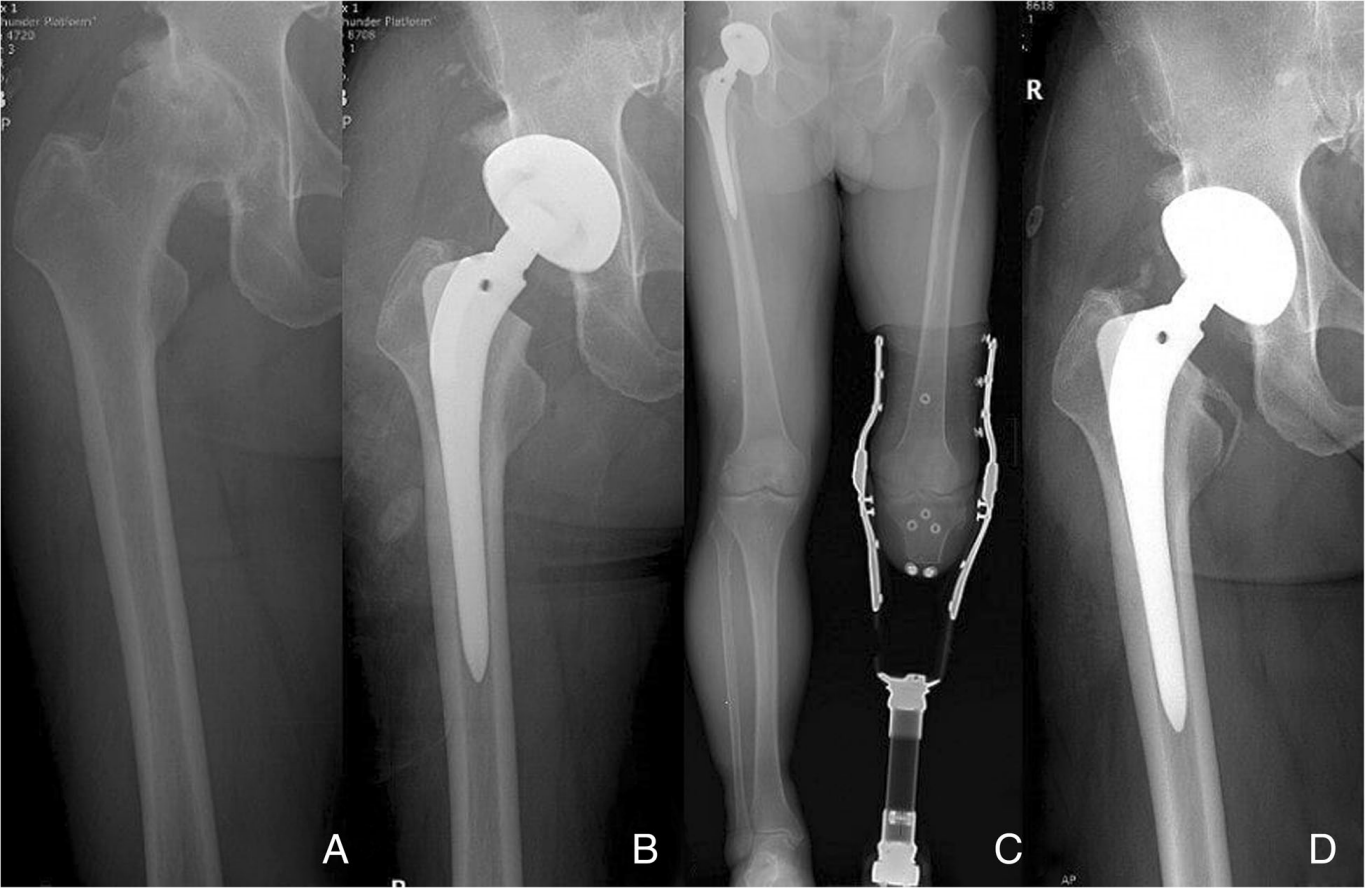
a Preoperative radiograph of a 67-old-year man with primary osteoarthritis of the hip joint. b Postoperative radiograph shows the excellent implant position of cementless total hip arthroplasty using the anterolateral approach. c Postoperative whole lower extremity radiograph of the prosthetic leg taken while standing. d At 7 years after the operation, the radiograph shows stable fixation of components without subsidence or changes in alignment
